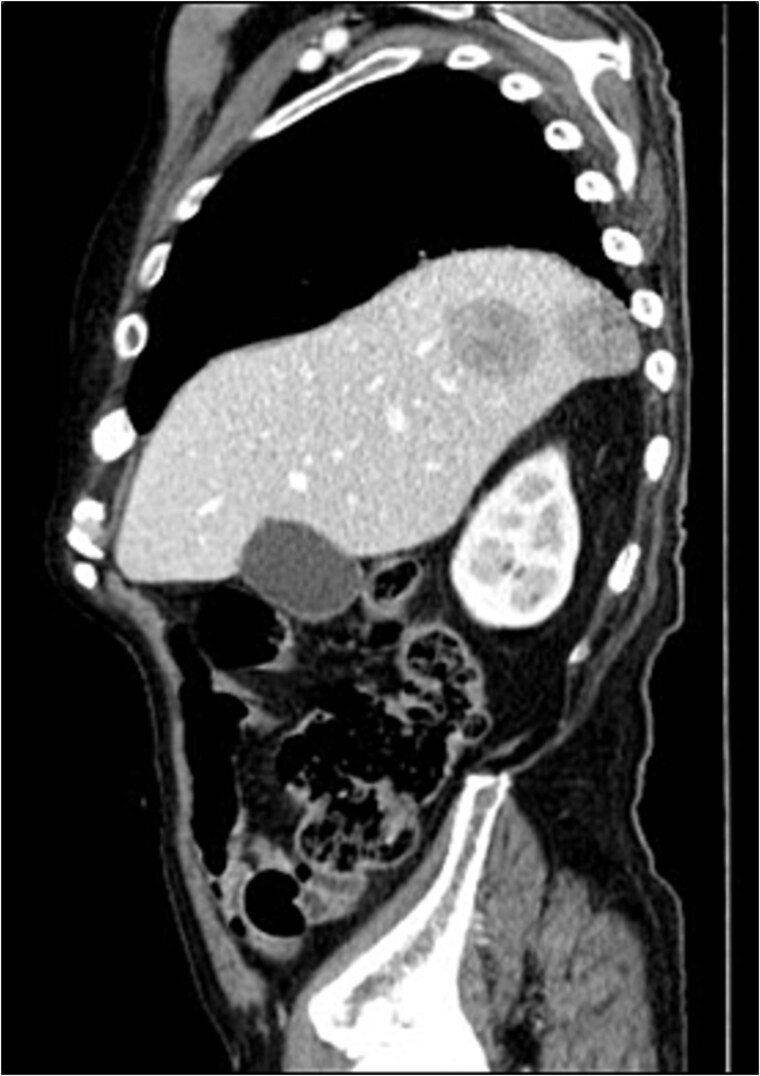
Liver metastasis (CT scan—Sagittal plane).
